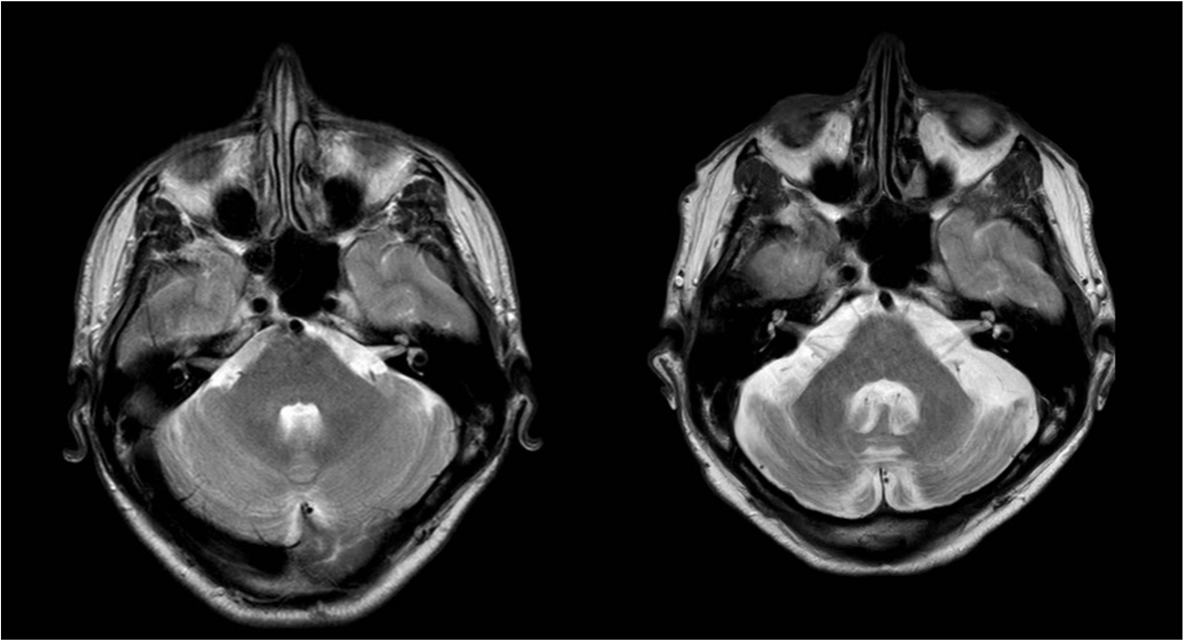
MRI (patient 3) at presentation (left) and 2 years later (right) showing the rapid development of cerebellar atrophy as a result of delayed diagnosis and treatment with gluten free diet and immunosupression
